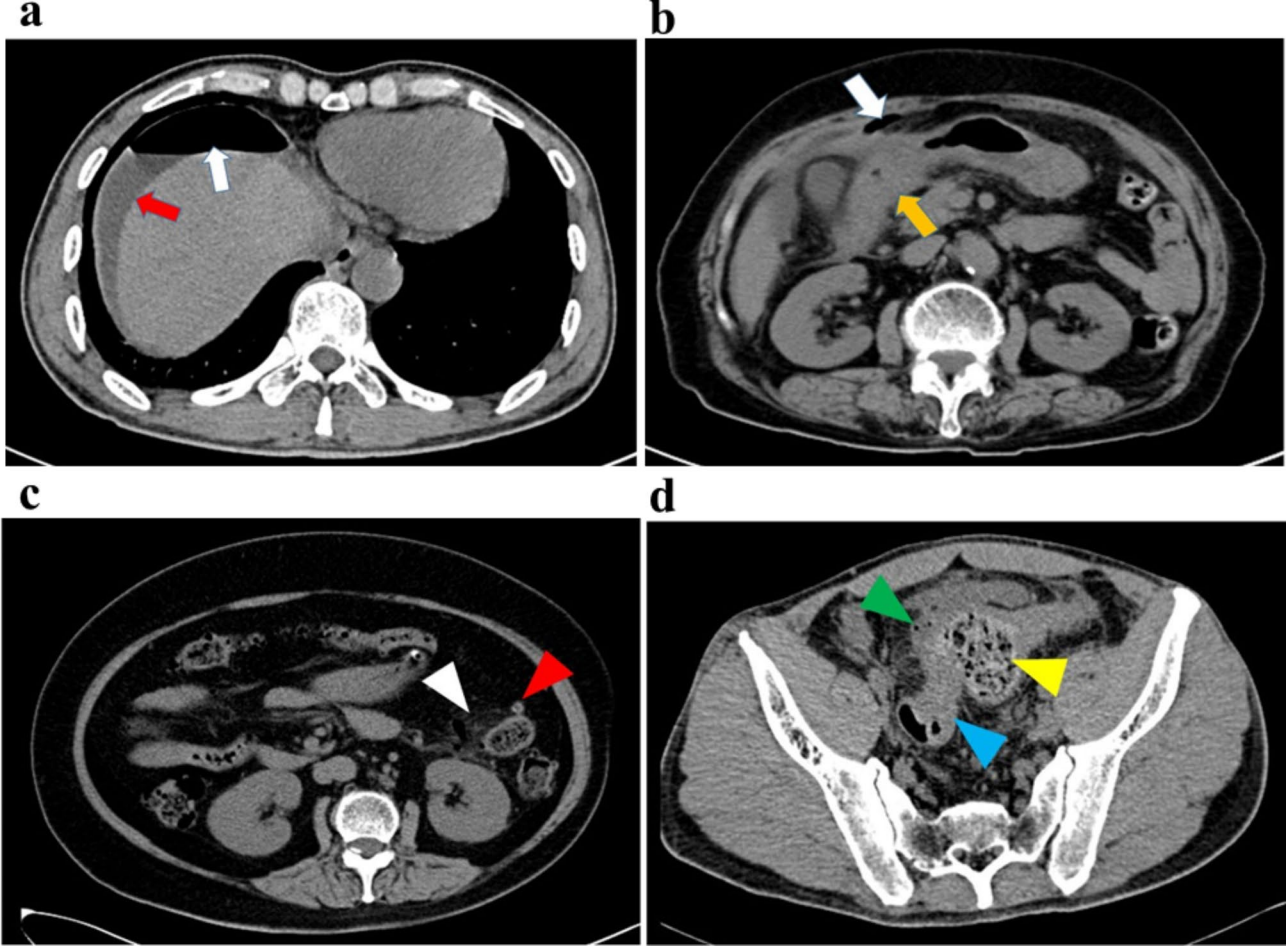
Abdominal CT for the patients (a) a 49-year-old man with perforation of the duodenal ulcer. Abdominal CT showed signs of subphrenic free gas (white arrow), perihepatic effusion (red arrow). (b) a 78-year-old woman with perforation of the duodenal ulcer. Abdominal CT showed signs of subphrenic free gas (white arrow), duodenal wall edema (orange arrow). (c) a 68-year-old woman with perforated diverticula of the descending colon. Abdominal CT showed inflammation and edema of tissues surrounding the descending colon (white triangle), diverticular lesion in intestinal wall (red triangle). (d) a 50-year-old man with perforation of the sigmoid carcinoma. Abdominal CT showed local bowel wall edema, stingy bubble (green triangle), carcinomatous lesion thickened, lumen stenosis (blue triangle), fecal retention proximal to the lesion (yellow triangle)
